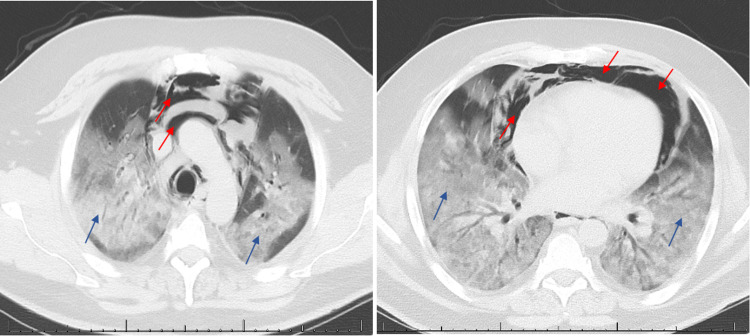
Chest CT scan demonstrating extensive pneumomediastinum (red arrows) with evidence of diffuse ground-glass opacities (blue arrows).
